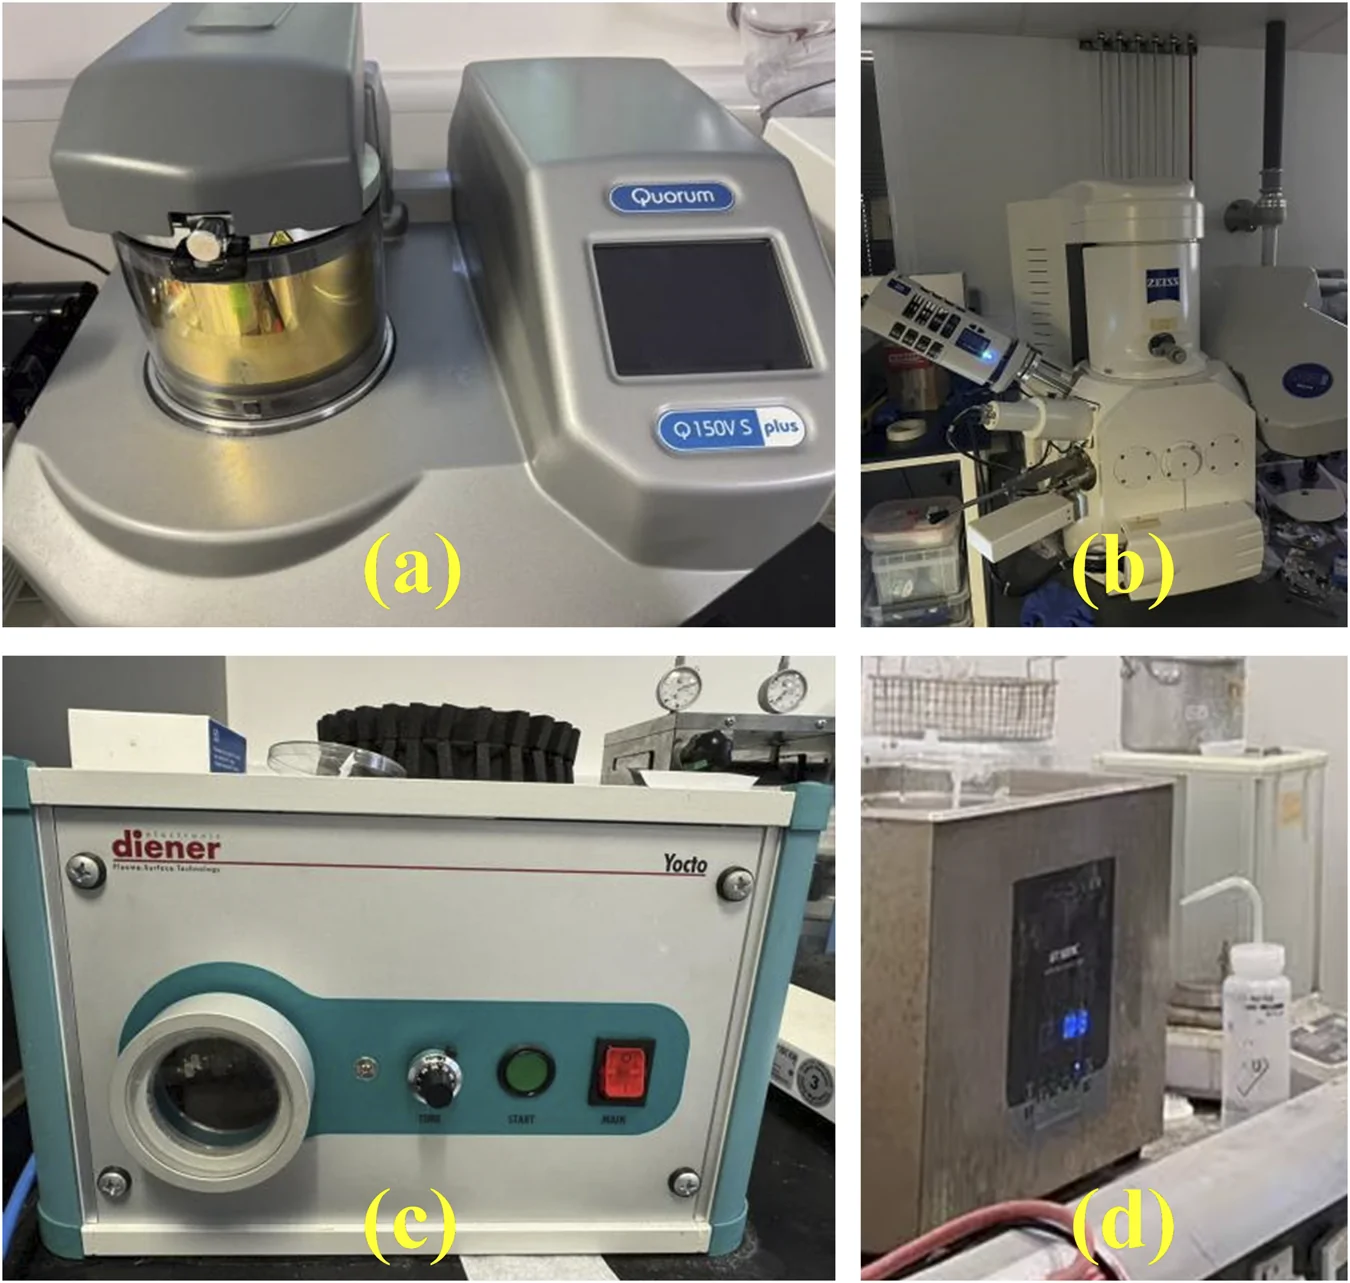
Experimental instruments for (a) Magnetron sputtering apparatus, (b) EDX and SEM, (c) Plasma cleaner and (d) Ultrasonic Cleaner.
